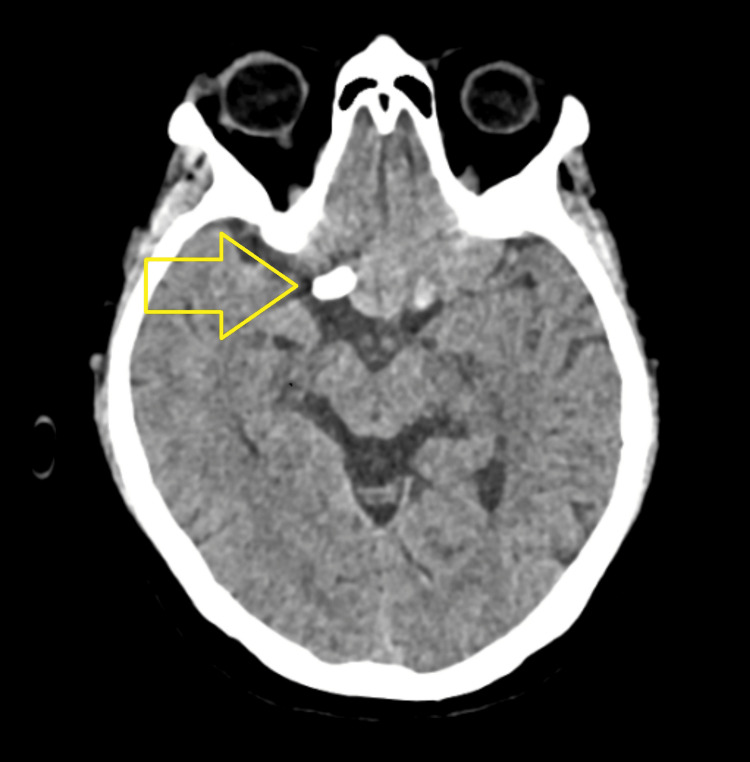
Axial non-contrast computed tomography (CT) of the brain at two-week follow-up demonstrating the flow-diverting device in situ in the suprasellar region, without evidence of complications (arrow).
